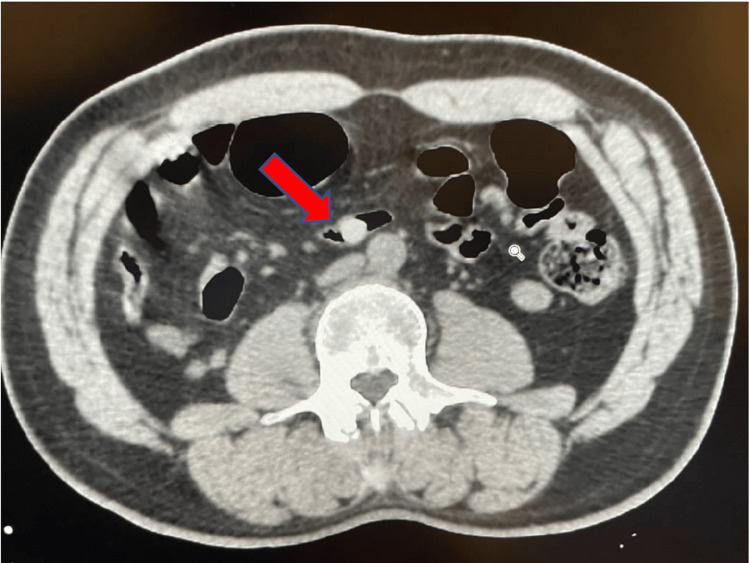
Computed tomography angiography showing hyperdense nodular formation in the ileum (arrow)
